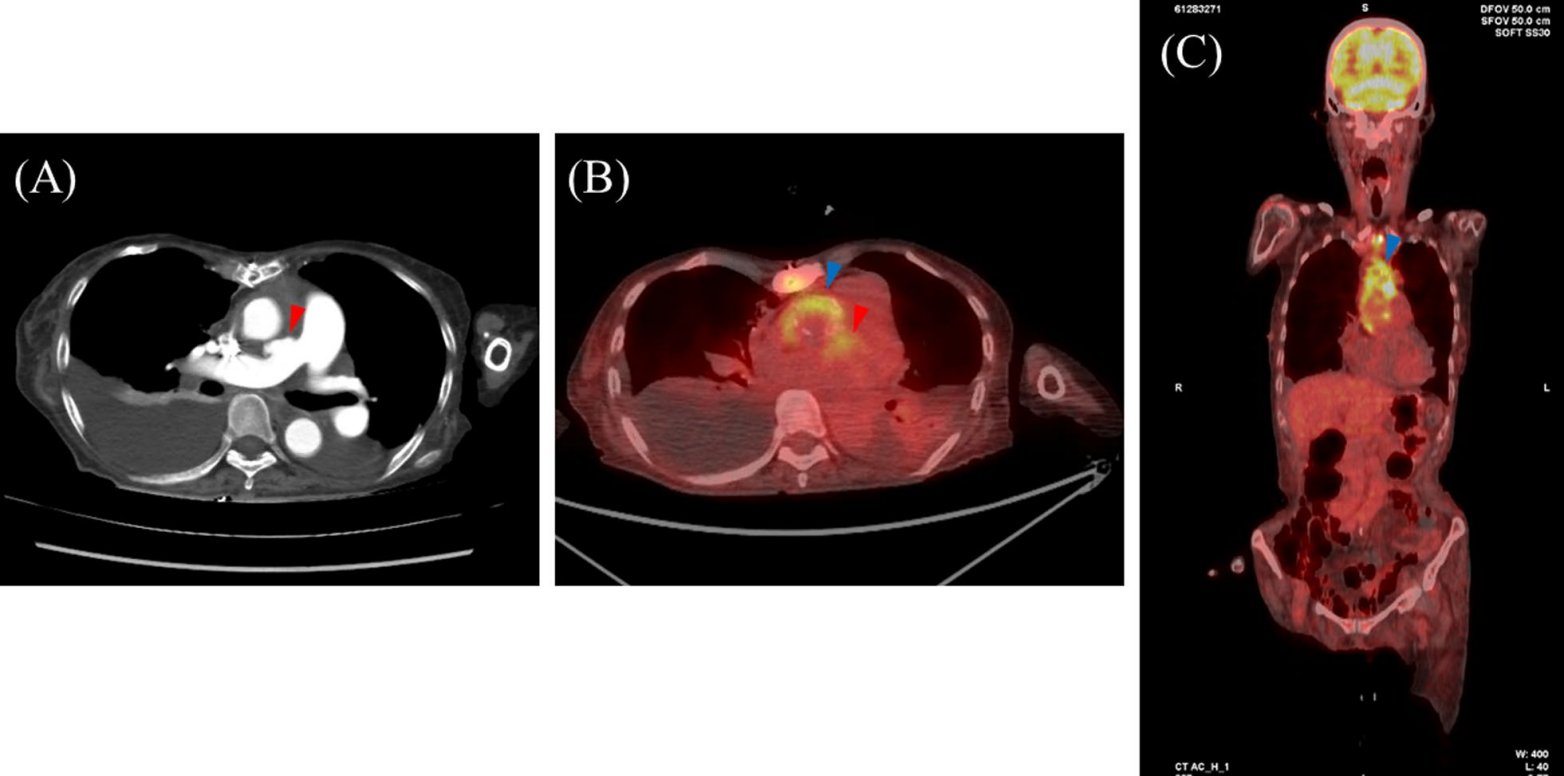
A Preoperative contrast CT showed a focal saccular outpouching from the right pulmonary artery (red arrowhead). B, C Preoperative FDG-PET showed focal uptake of FDGs along the PAP (red arrowhead) and ascending-arch graft (blue arrowhead)
